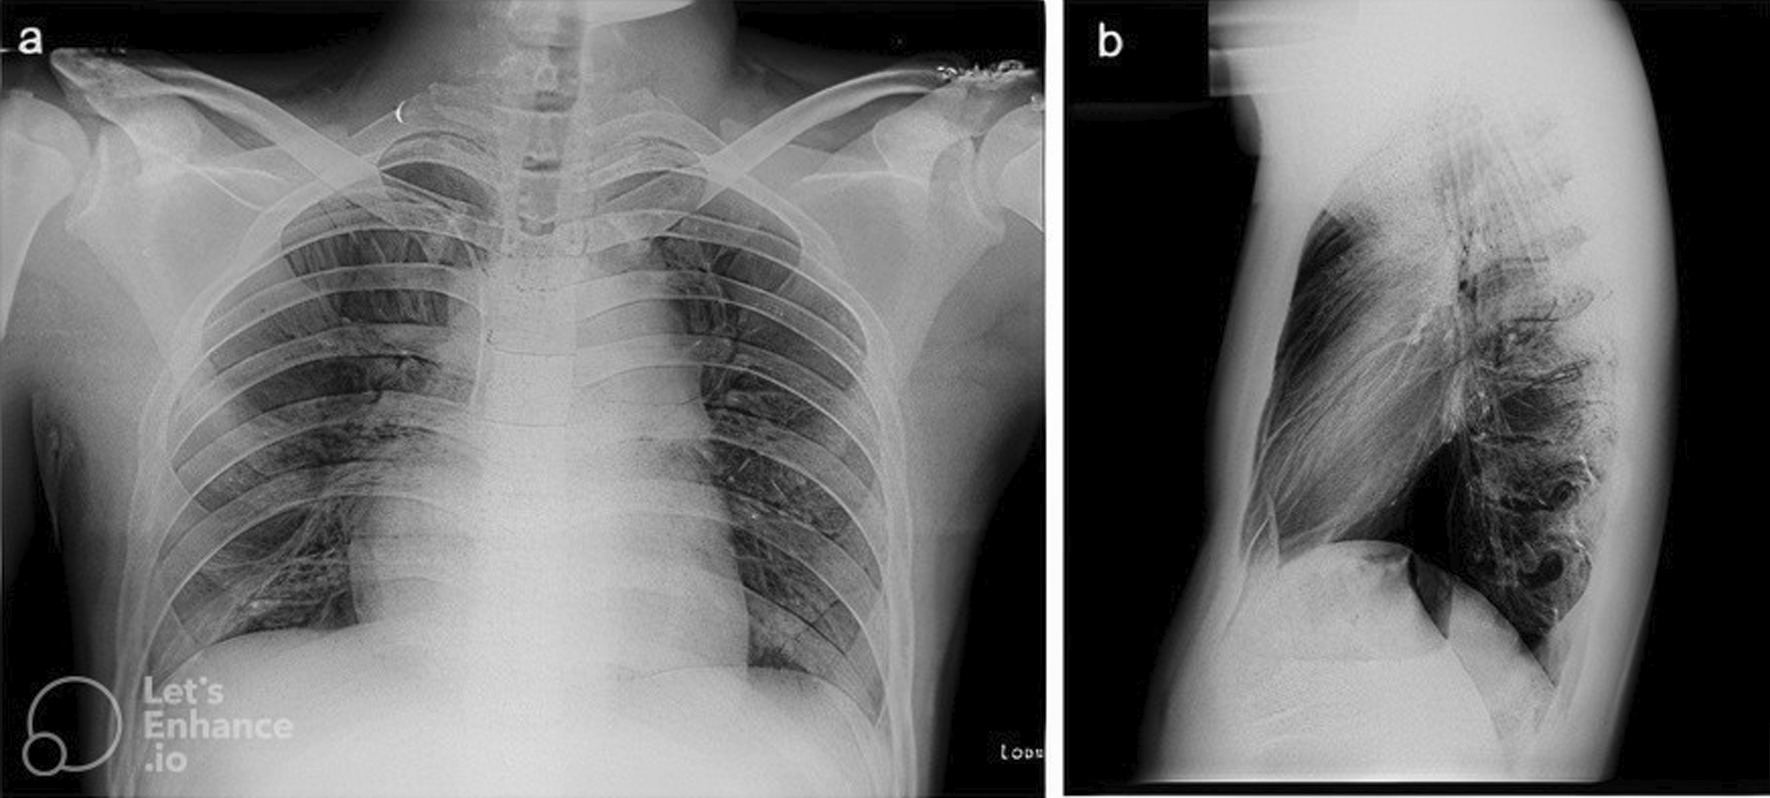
Patient’s normal chest X-ray. A Posteroanterior (PA) view. B Lateral view
